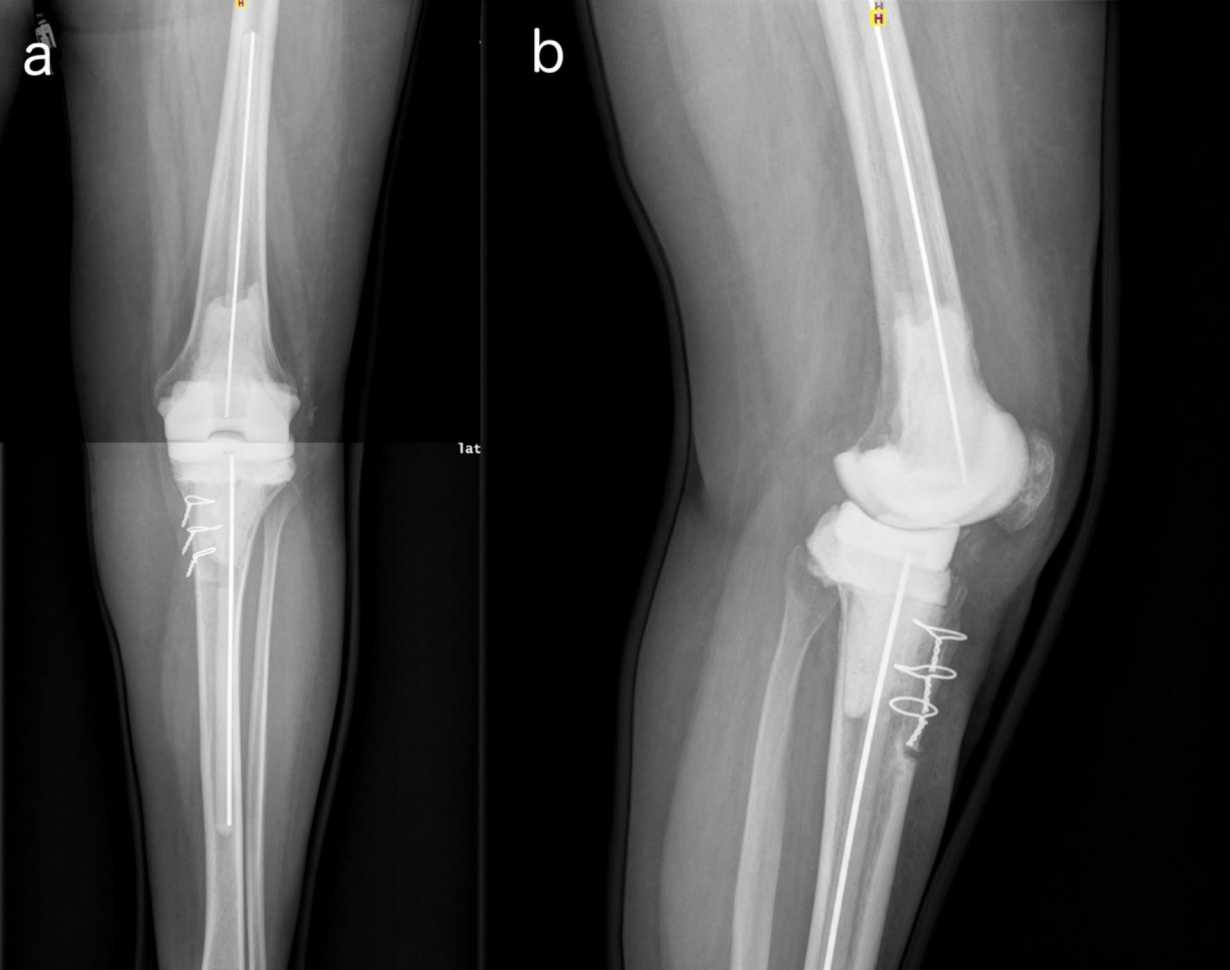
a Anteroposterior and b lateral radiography of a vancomycin–gentamicin prefabricated antibiotic cement spacer (Vancogenx®, Tecres, Sommacampagna, Italy). In some cases, aiming to increase stability and to fill the intramedullary dead space, a hand-made antibiotic-loaded cement stem (reinforced with a Steinmann pin) is connected to the spacers as can be seen in the radiographies
